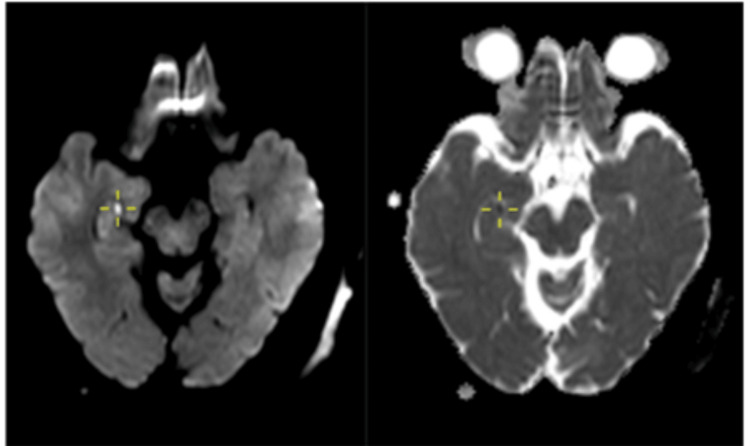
Case 2: Image on the left shows a punctate DWI lesion involving the right hippocampus with a corresponding area of low ADC on the right image (cross-marked). DWI: diffusion-weighted imaging; ADC: apparent diffusion coefficient.
